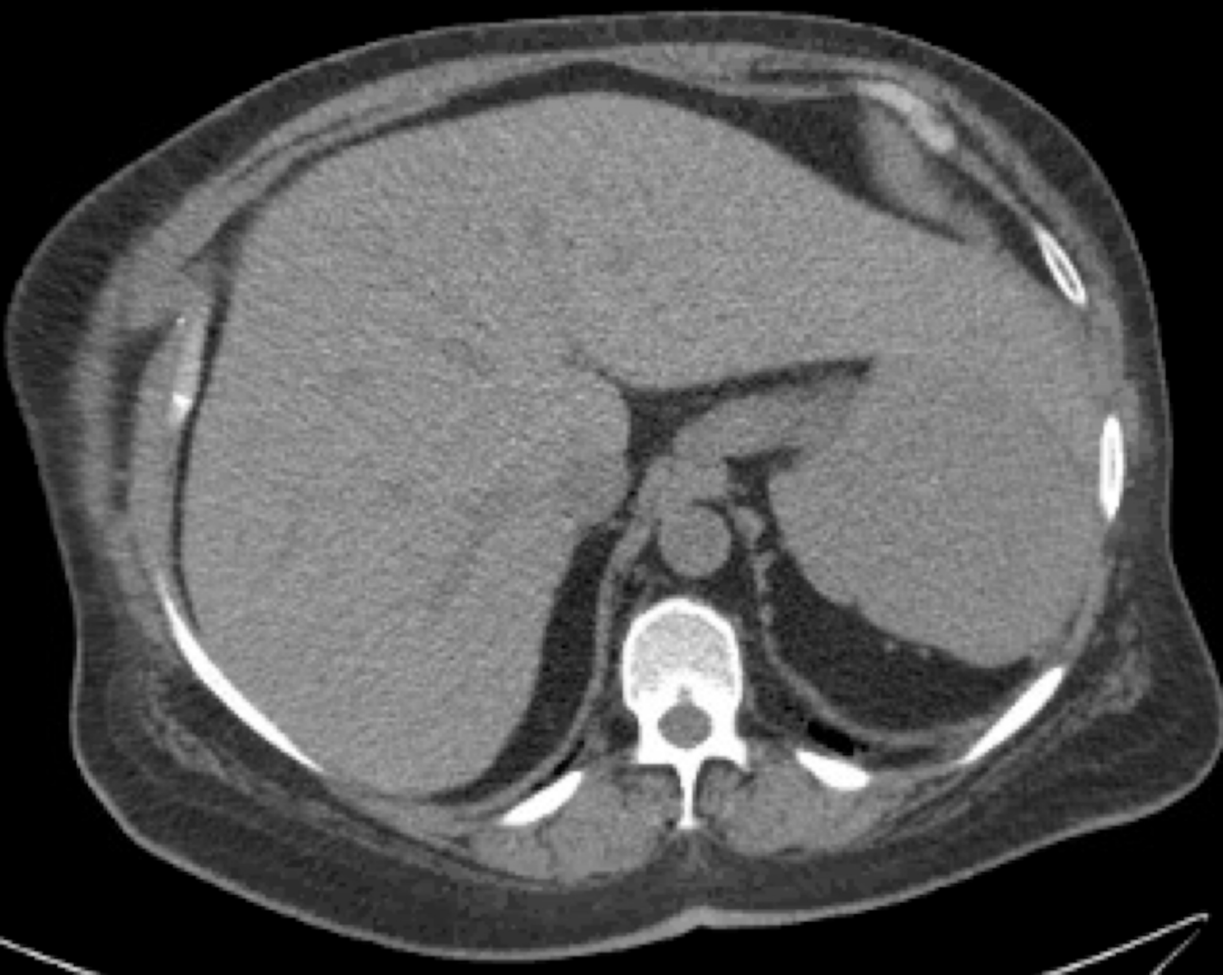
Computed tomography (CT) of the abdomen did not reveal any abnormality
